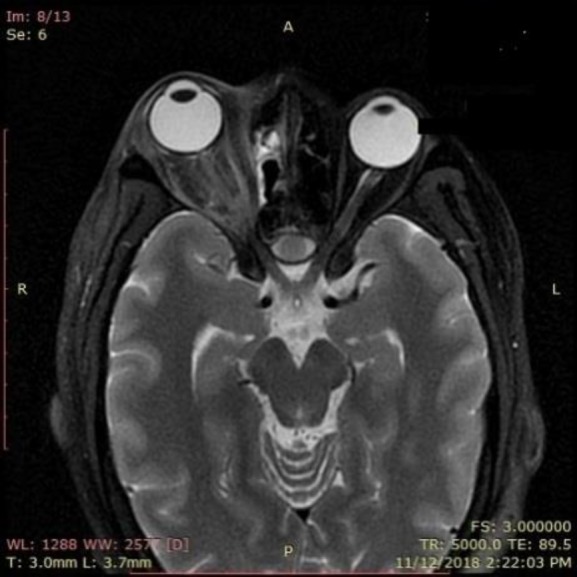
Contrast-enhanced magnetic resonance image showing fungal rhinosinusitis, along with proptosis of the right eye
